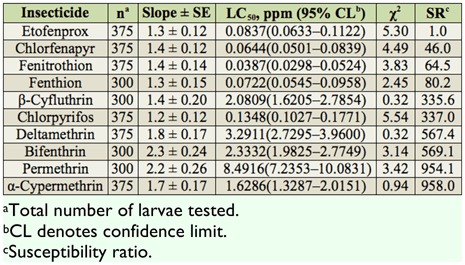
Summary of toxicities of 10 insecticides to third instars of the JJ-CT strain of *Culex tritaeniorhynchus* based on the 24 hr exposure contact mortality bioassays.
